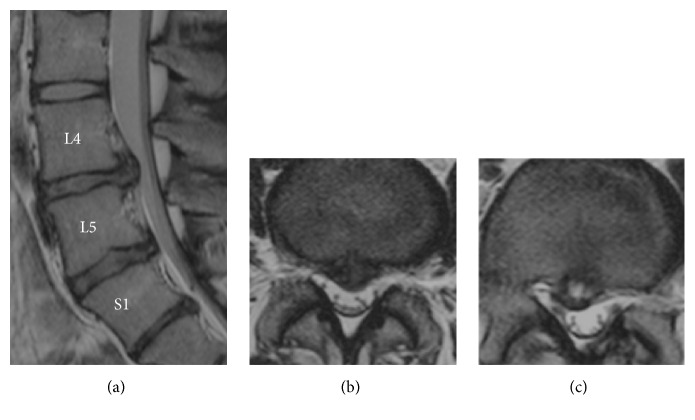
MRI showed posterior lumbar disc herniation connecting the nucleus pulposus tissue at L4/5 at the age of 18 years ((a) and (b)). The lumbar disc herniation at L5/S1 was absorbed, although the posterior endplate lesion still remained at L5/S1 ((a) and (c)).
